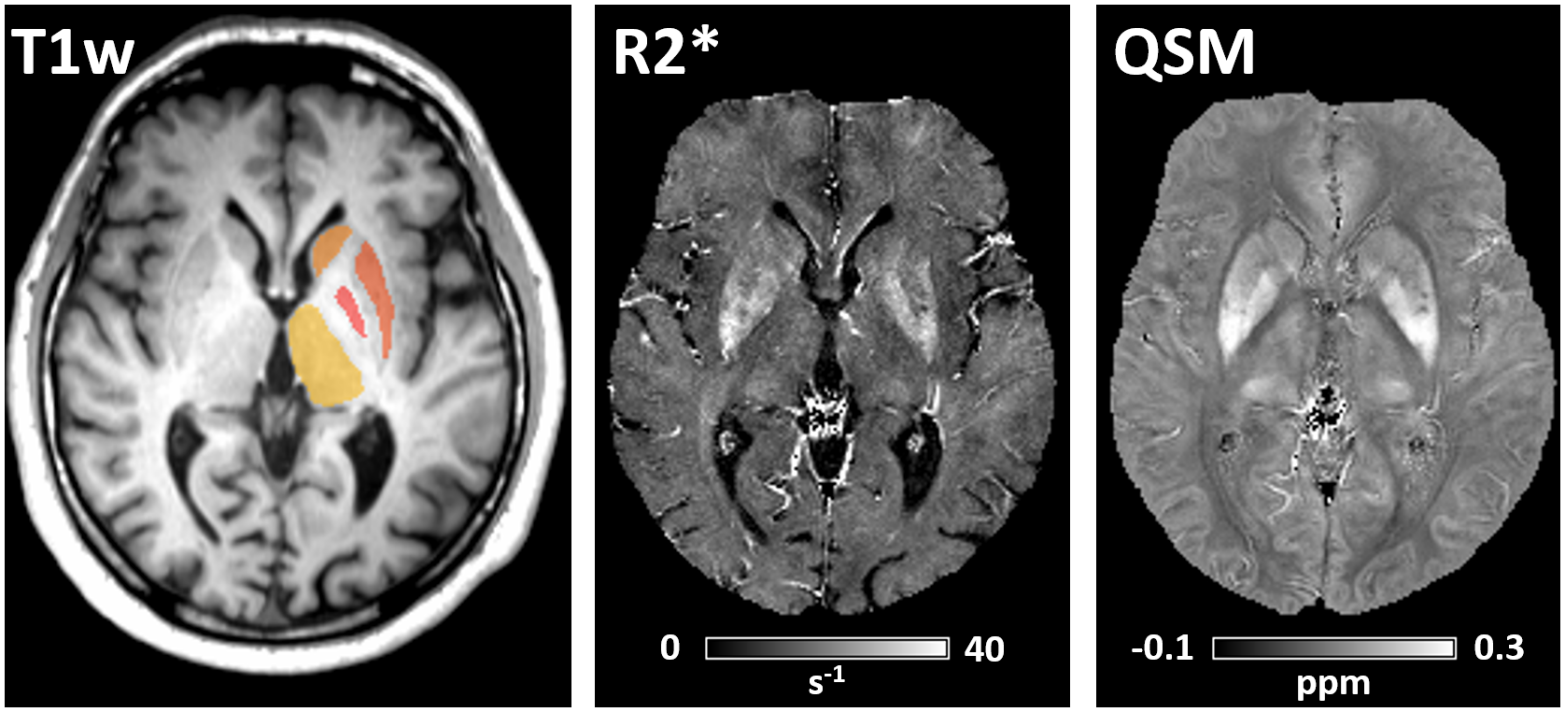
Representative T1-weighted MP-RAGE image (left) with color overlay of segmented deep gray matter structures, R2* relaxation rate constant map (center) and quantitative susceptibility map (right) of a 62-years patient with PD (MDS-UPDRS 46; Hoehn & Yahr 2). High R2* rates and paramagnetic susceptibilities are found in the basal ganglia. Note the improved gray-white matter contrast on the QSM map compared to the R2* map.
